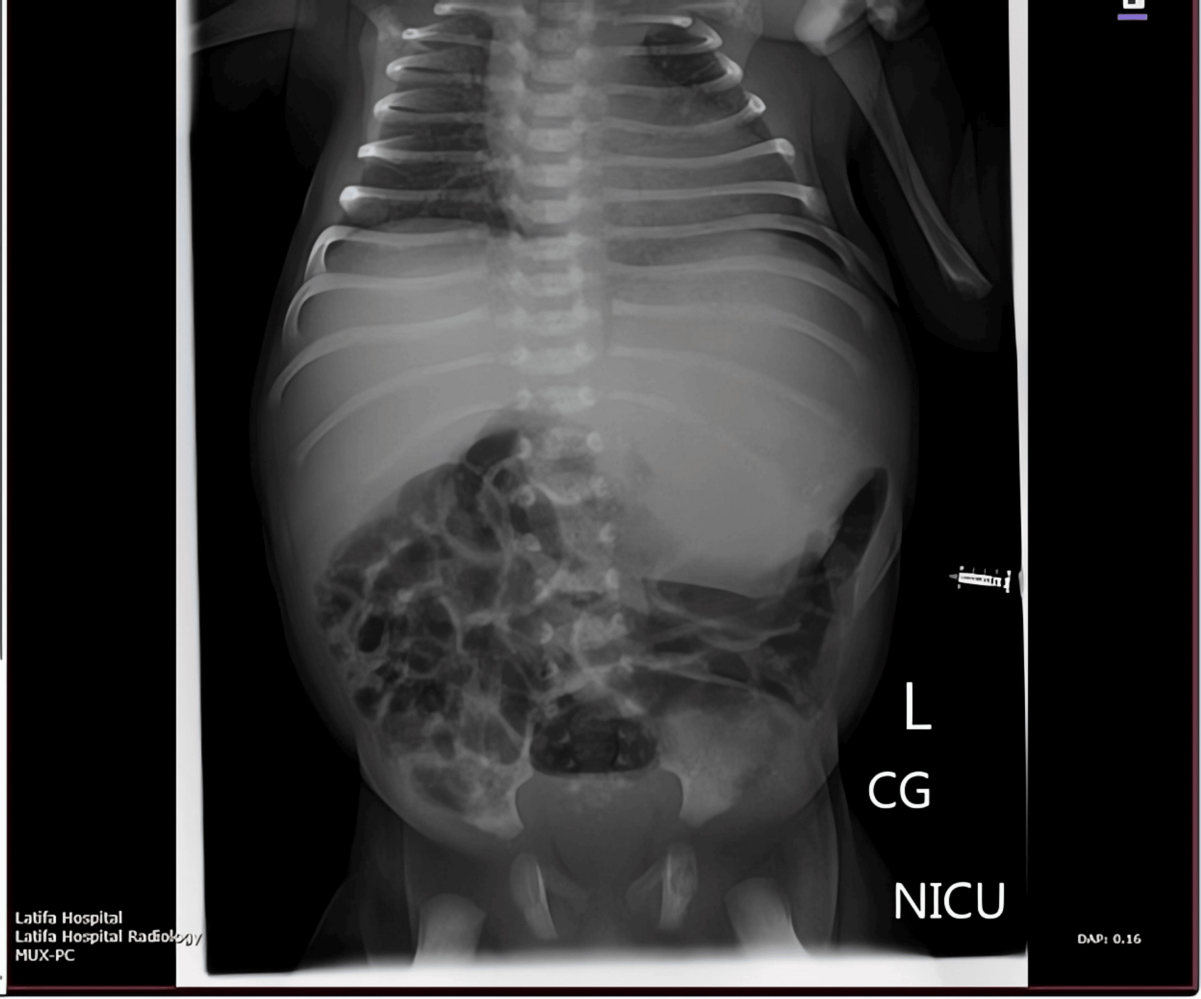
Day 8 radiograph showing emerging calcified epigastric mass Follow-up abdominal radiograph on day 8 of life, showing more defined gastric silhouette and progressive downward displacement of bowel loops. Coarse calcifications are visible overlying the mass in the left upper quadrant.
